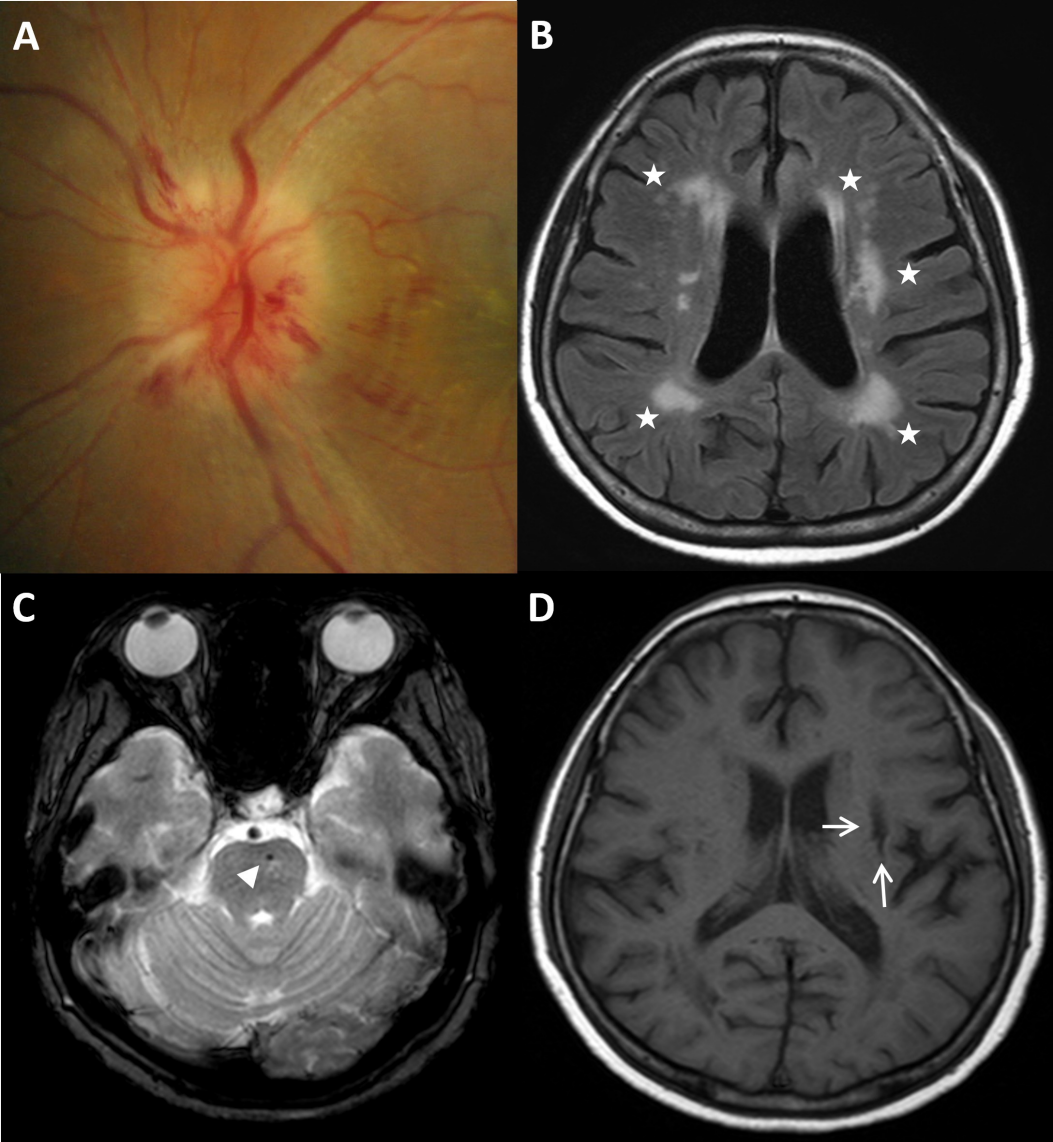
Cerebral small vessel disease in a patient with NAION. (A) A case of NAION with hyperemic disc, segmental swelling, and disc hemorrhage in a 62-year-old woman. (B) White matter hyperintensities (Fazekas grade 3) on brain MRI (FLAIR image: white asterisks). (C) Cerebral microbleeds on T2-weighted brain MRI (white arrowhead) (D) silent lacunar infarct on T1-weighted MRI (white arrow) corresponding to cerebral small vessel disease. NAION: nonarteritic anterior ischemic optic neuropathy; MRI: magnetic resonance imaging; FLAIR: fluid attenuated inversion recovery.
